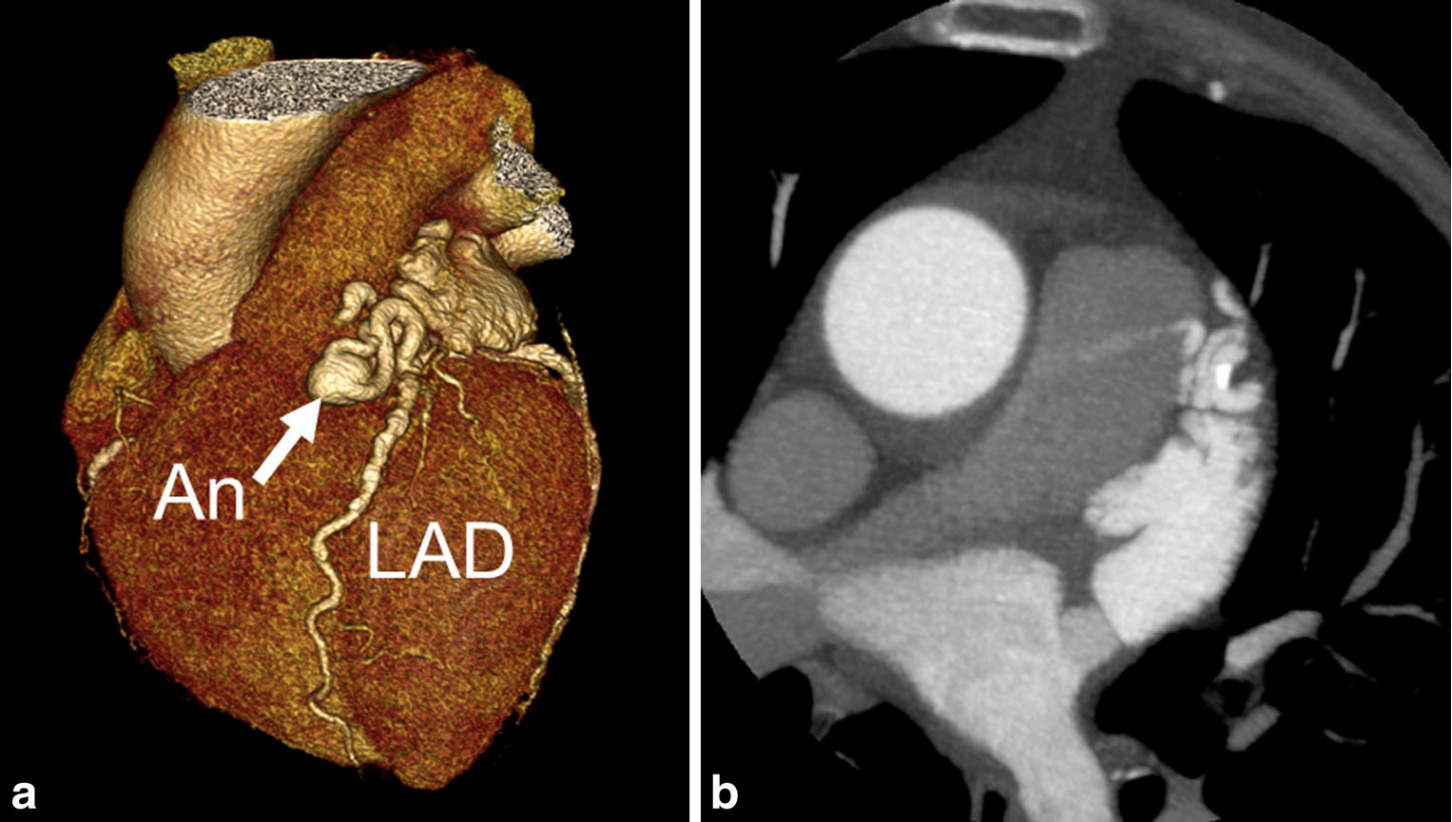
A 75-year female patient with a history of hypertension and type 2 diabetes mellitus presented with atypical complaints resulting in syncope. Echocardiography was suggestive for a coronary artery fistula to the pulmonary artery. (a,b) Four-mm diameter fistula, (a) volume rendering and (b) MIP image, arising from the proximal part of the left anterior coronary artery (LAD), with a thirteen-mm diameter aneurysm (An) within the fistula. (b) The fistula ends at the main pulmonary artery, note the small contrast jet from the fistula into the pulmonary artery (arrow). Patient had atherosclerotic plaques in the coronary arteries without significant stenosis
